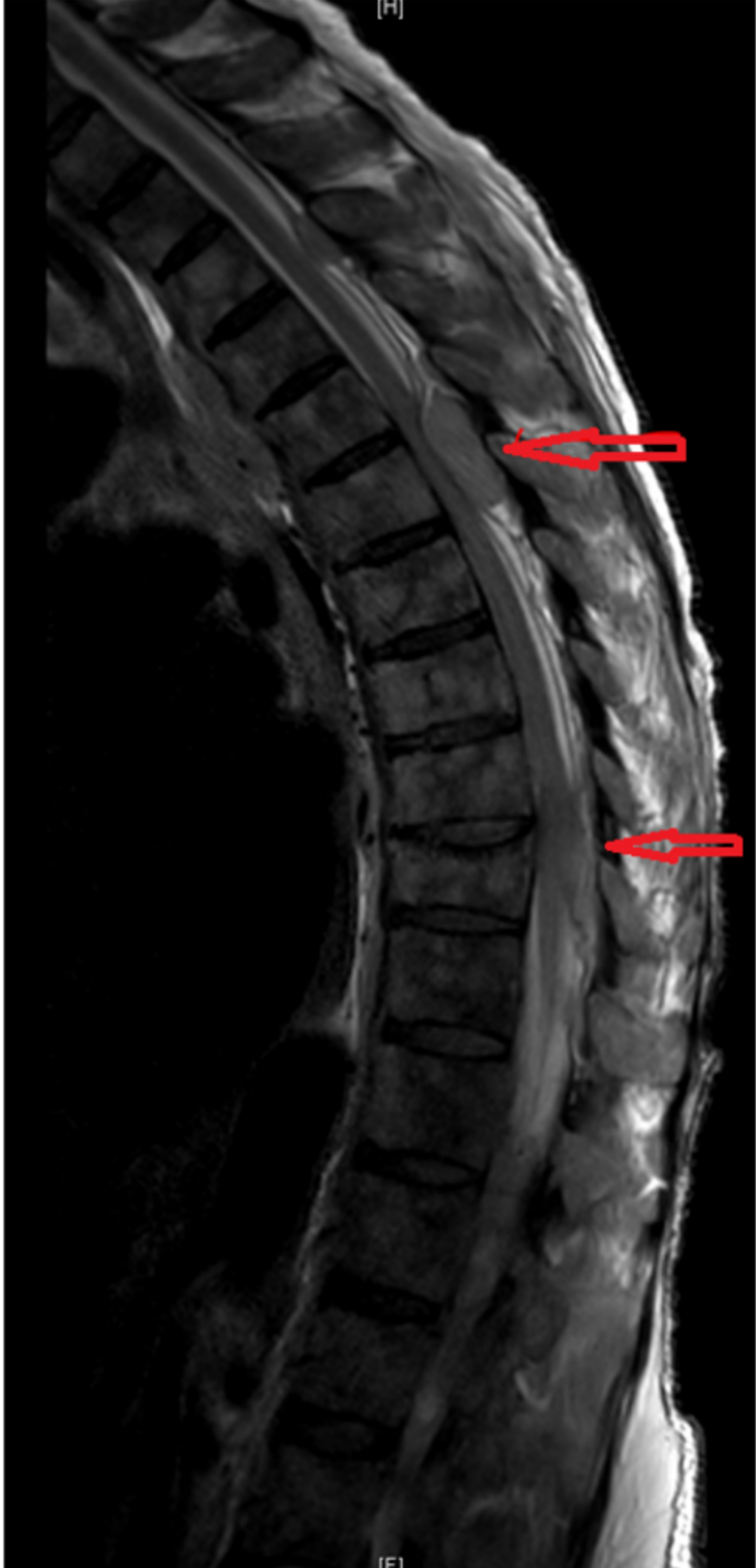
MRI of thoracic spine-posterior epidural soft tissue in the thoracic spine at T3-T4, T5/T6/T7, and T9-T10 with resultant cord compression most prominent at T6 and T9.
